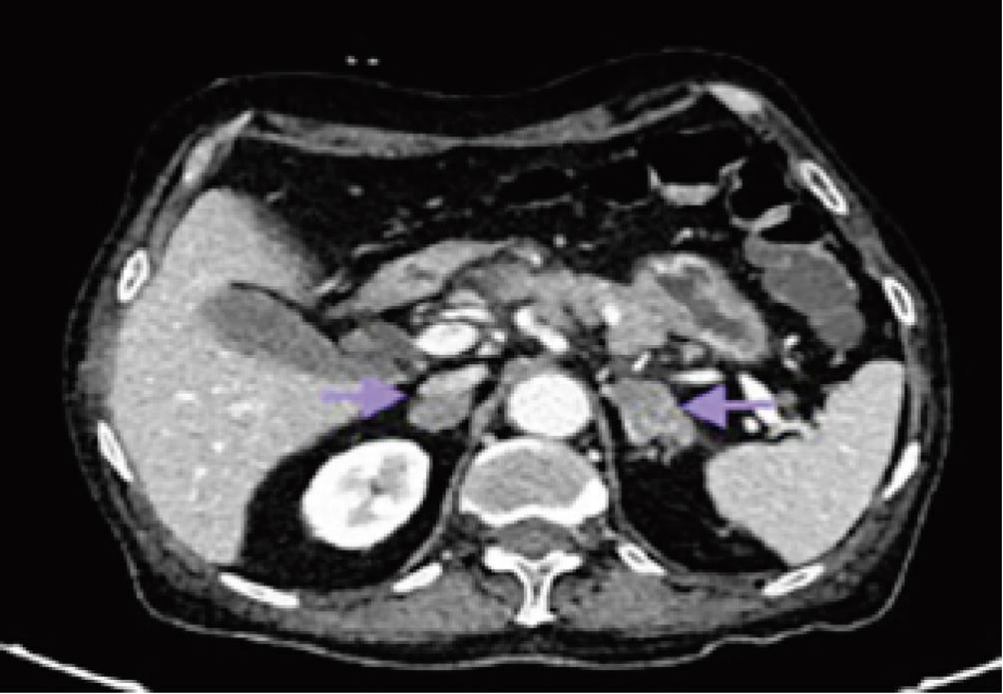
Contrast‐enhanced computed tomography of the abdomen showing metastatic bulky bilateral adrenal glands (arrows).
